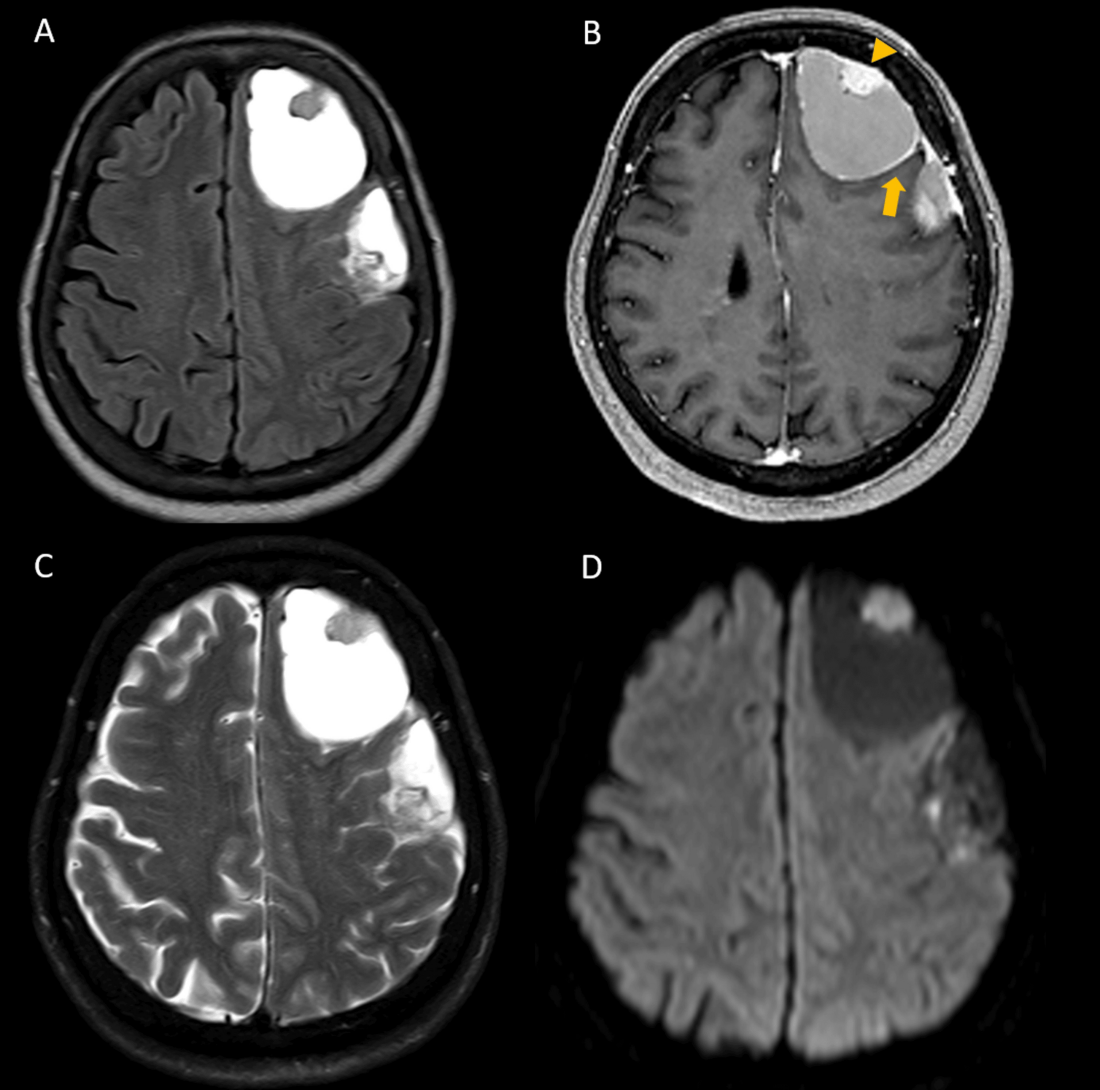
Brain MRI images of case 2 Two extra-axial cystic lesions, measuring 6.5 and 5.7 cm, respectively, with hyperintensity signal on both T1 (A) and T2-weighted images (C). Focal contrast enhancement (yellow arrowhead) was noted at the mural nodules and mild enhancement at cyst walls (B, yellow arrow). Corresponding hyperintense signal changes on diffusion-weighted imaging (DWI) were observed at these enhancing sites (D).
